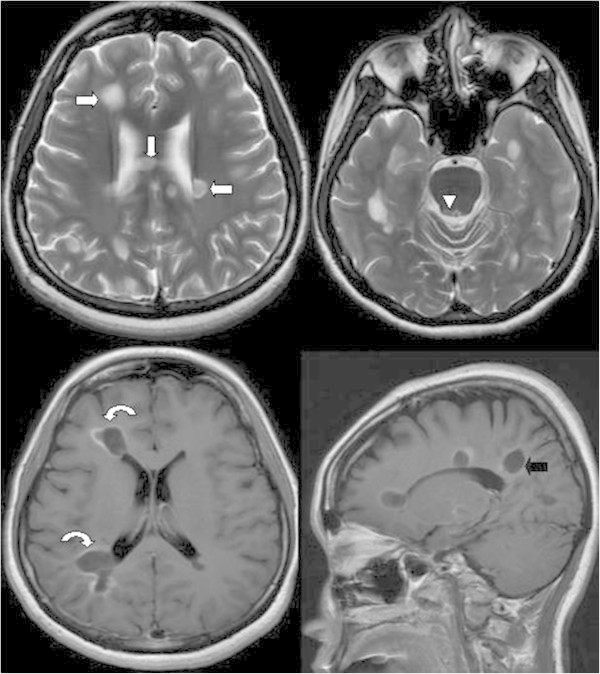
Two months later, the hyperintense lesions located in the bilateral periventricular white matter (arrow), body of the corpus callosum (arrow), and right periaqueductal grey matter (arrowhead) were increased in number and size, as observed on T2-weighted images. After gadolinium contrast injection, more prominent ring and incomplete ring enhancement over these lesions is noted on the T1-weighted image (curved arrows). In the sagittal view, multiple sclerosis plaques with an increased number of Dawson’s fingers are noted (black arrow).
